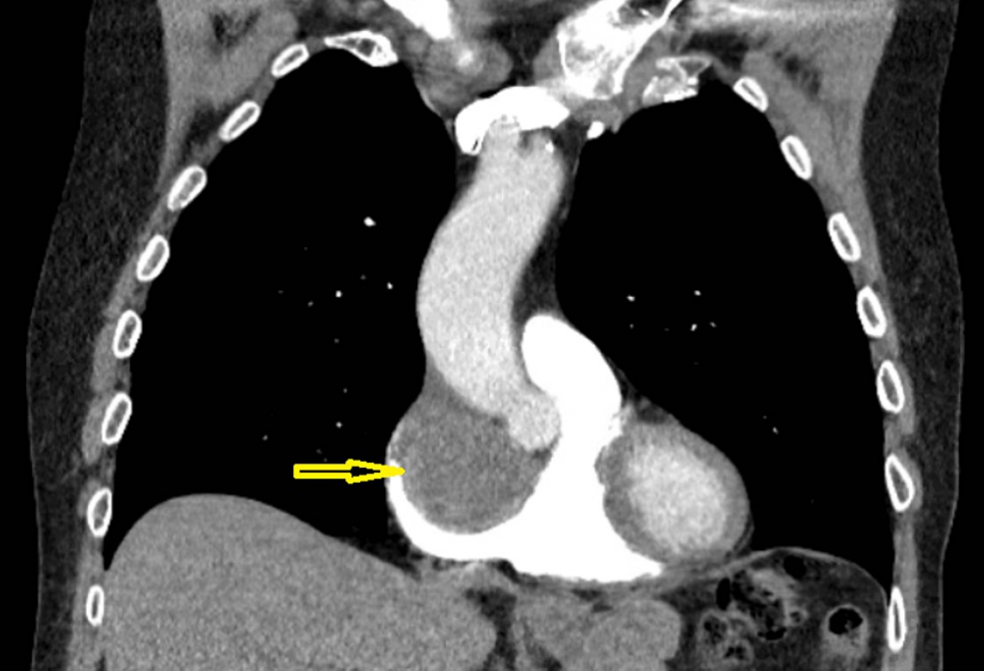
Computed tomography angiogram for pulmonary embolism. Yellow arrow pointing to contrast filling defect at the location of the right atrial thrombus.
